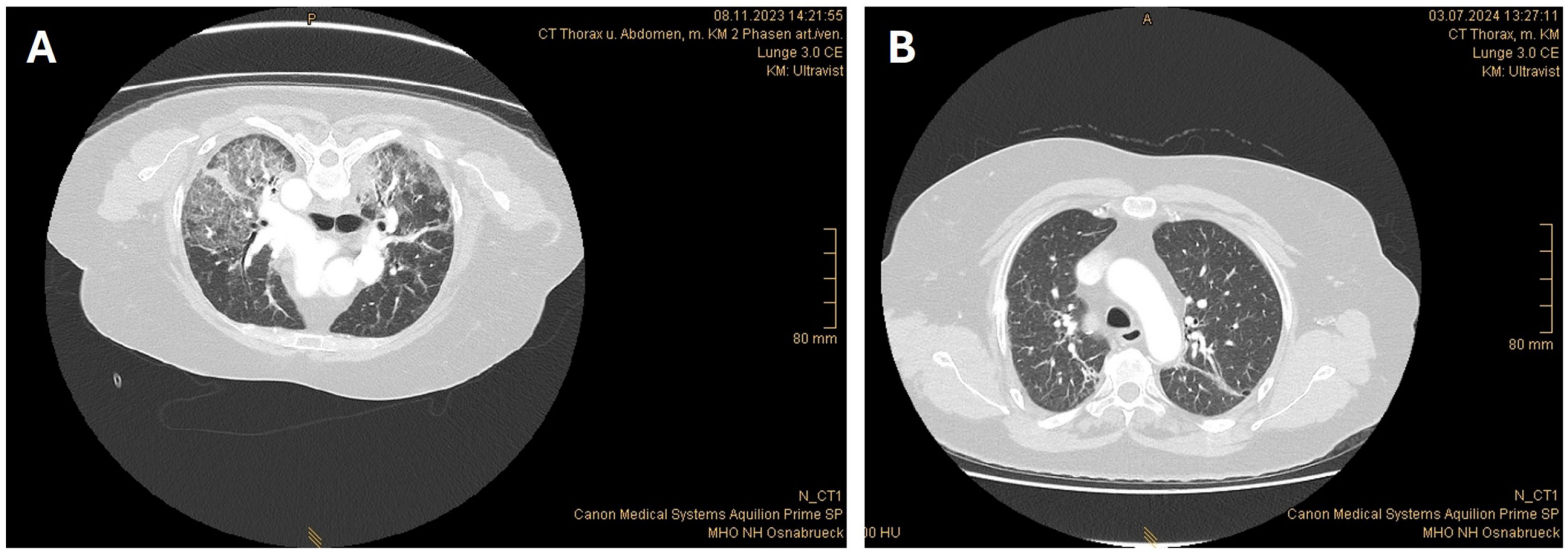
Thorax CT shows bilateral extensive interstitial pneumonia before ravulizumab initiation (A) and reduced pulmonary infiltrates, residual consolidation from resolved pneumonitis, as well as suspected encapsulated effusions after ravulizumab initiation (B).
